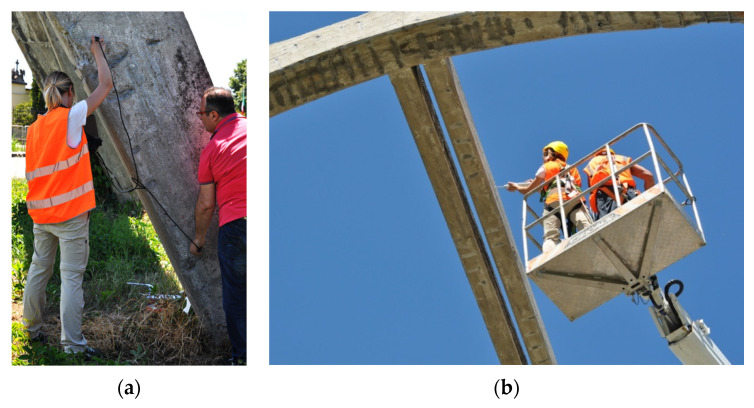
On-site testing of the various elements of the structure: (a) ultrasonic pulse velocity test; (b) rebound hammer test on one of the top beams connecting the arches.
